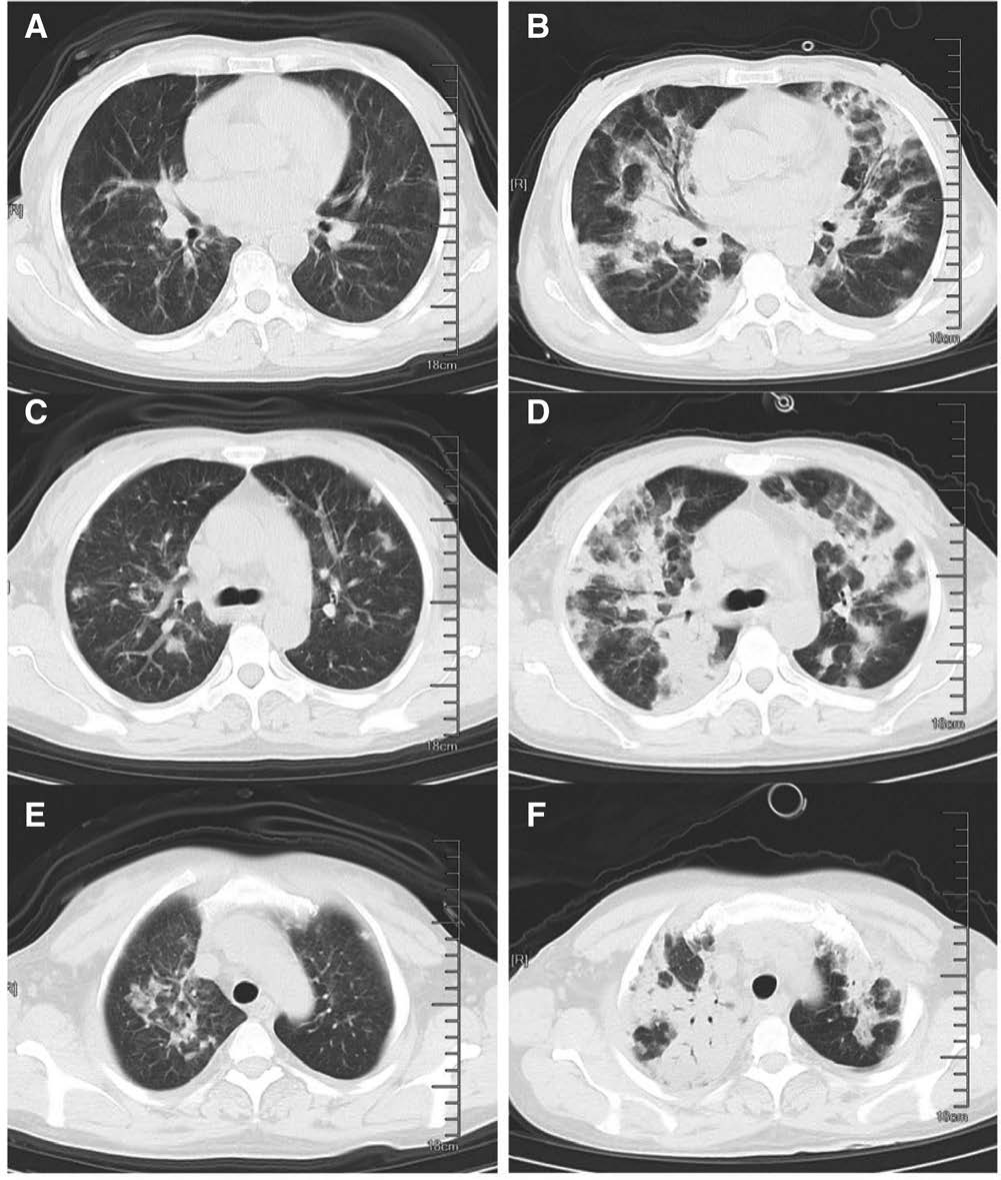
Chest CT-scan on the day 5 (A, C, E) and day 9, (B, D, F) of admission. Bilateral lung exudation and the range was significantly larger and the focus was denser than before. CT = computerized tomography.
